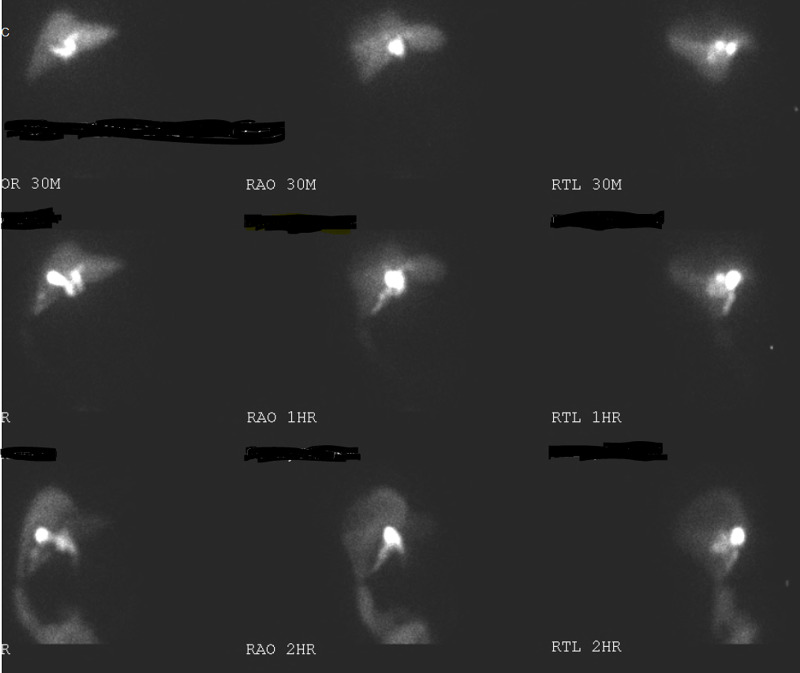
Hepatobiliary iminodiacetic acid (HIDA) scan showing no scintigraphic evidence of biliary obstruction, delayed visualization of gall bladder and bowel may represent chronic cholecystitis, patent common bile and cystic duct, and tracer accumulating along the right abdomen
